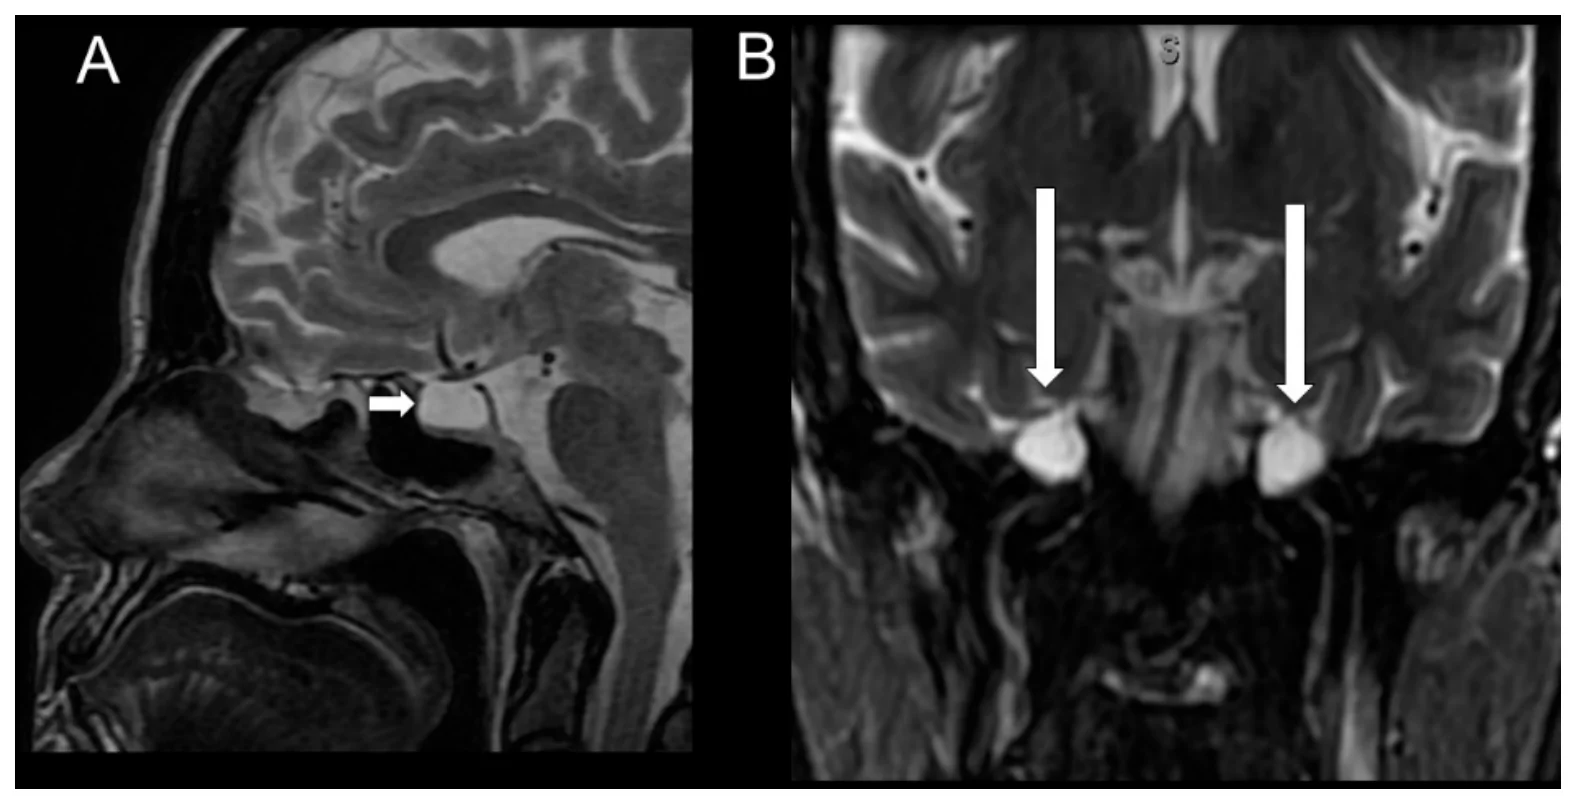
T2 weighted sagittal (A) and coronal (B) MRI scans demonstrating an empty sella (short arrow) and dilated Meckel’s caves (long arrows).
